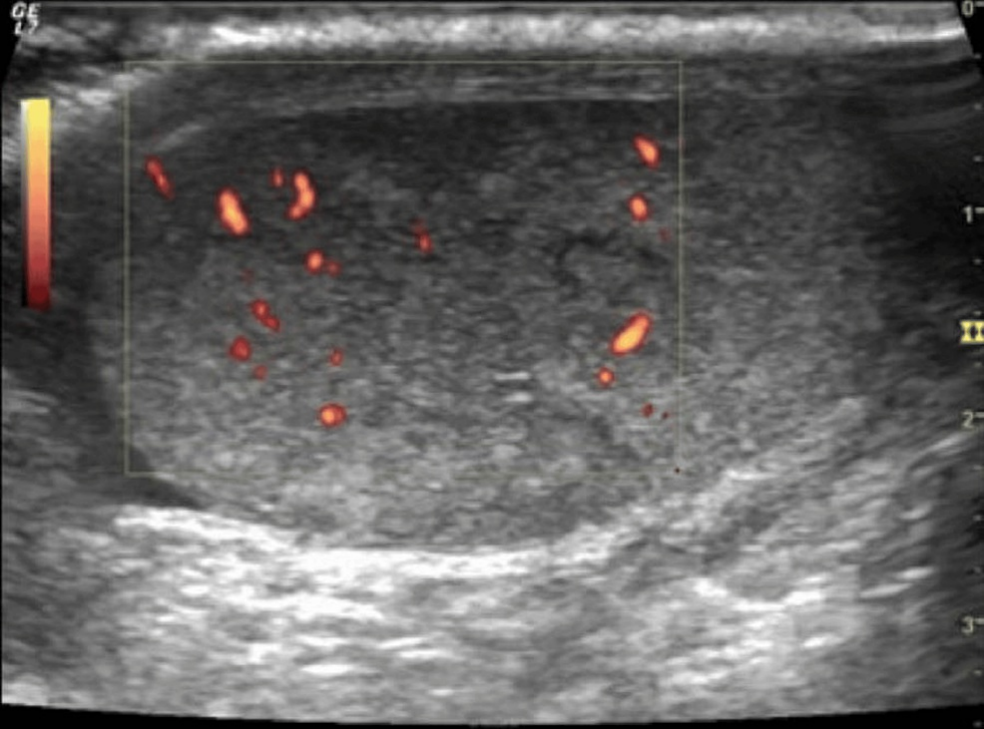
Scrotal ultrasound Heterogeneity in the left testis, with pseudo-nodular areas
